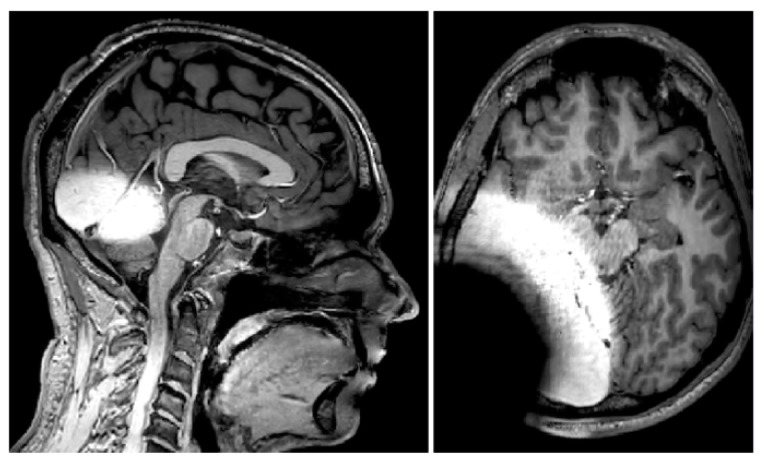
Visualization of the MRI artefacts caused by a unilateral cochlear implant (CI). In the left panel, the midsagittal plane has been visualized, showing large artefacts around the temporal lobe, while the inter-hemispherical corpus callosum is not hampered. The right panel shows an axial slice with extensive MRI artefacts.
